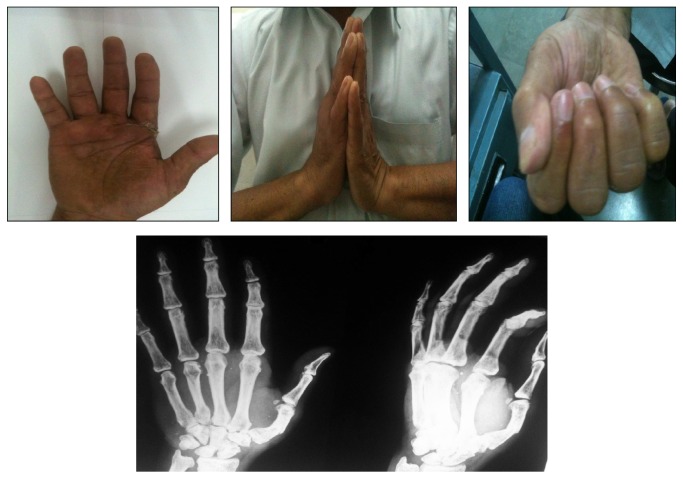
Clinical picture and X-ray at 3 months.
